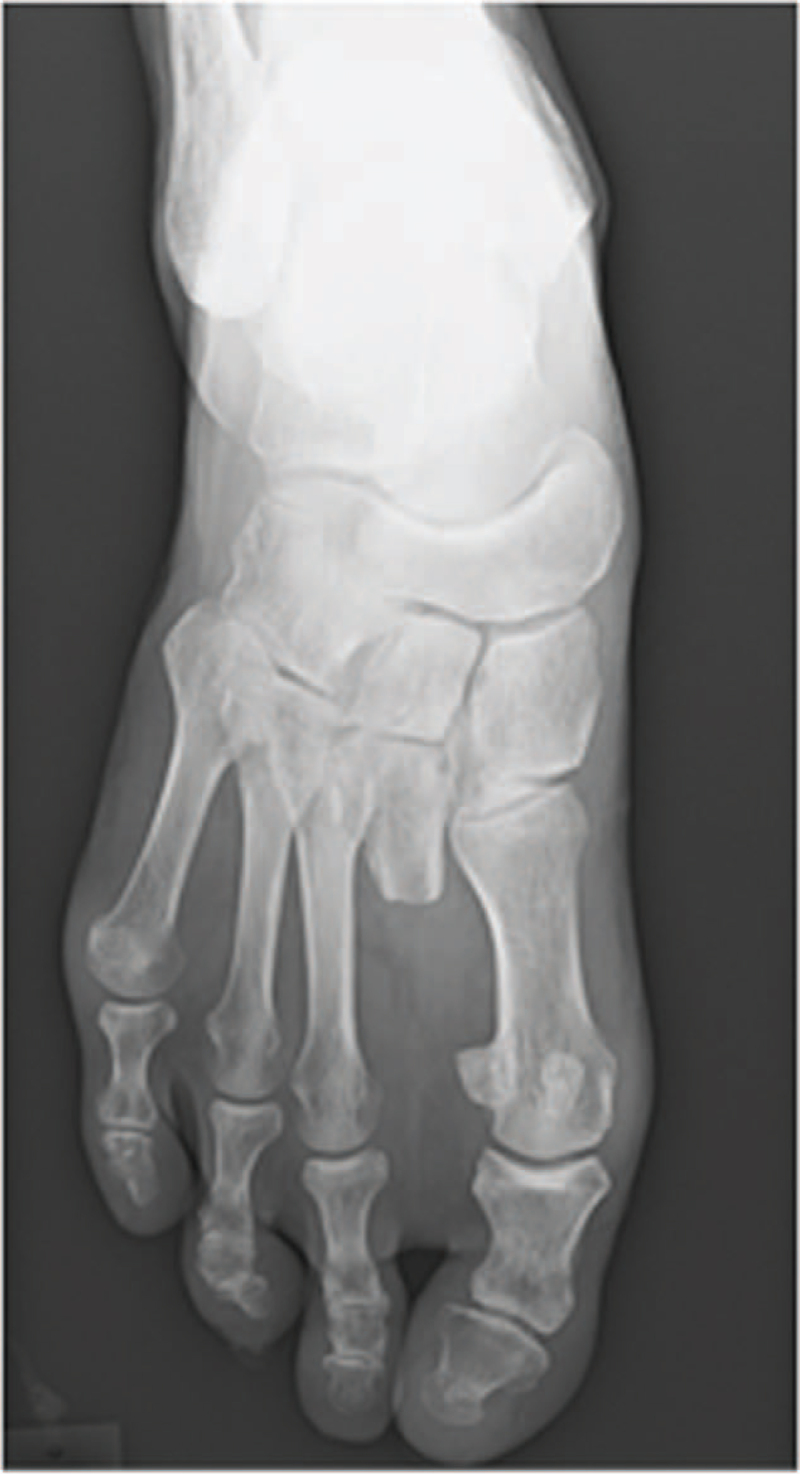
The radiograph of the right foot after the surgical treatment.
